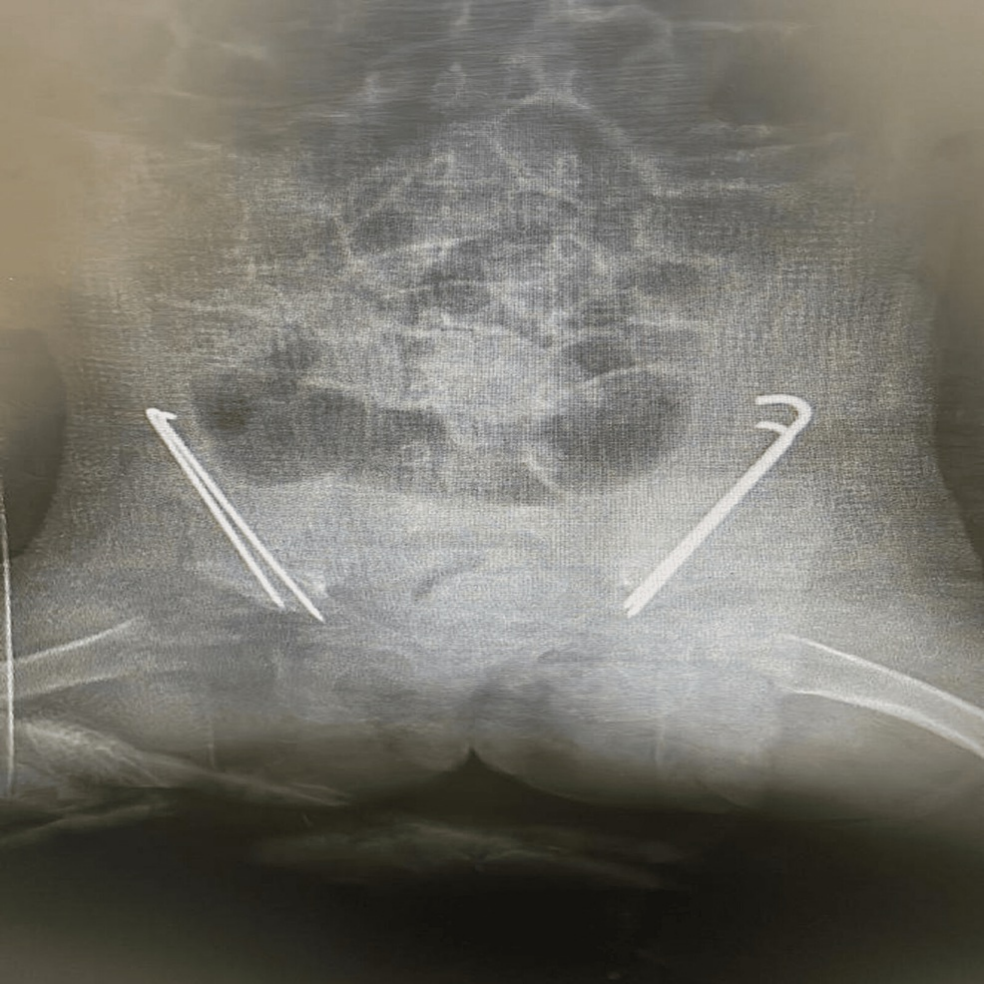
A four-year-old DDH patient who underwent femoral and pelvic osteotomy. The X-ray was obtained from one of the patients in the clinic.
